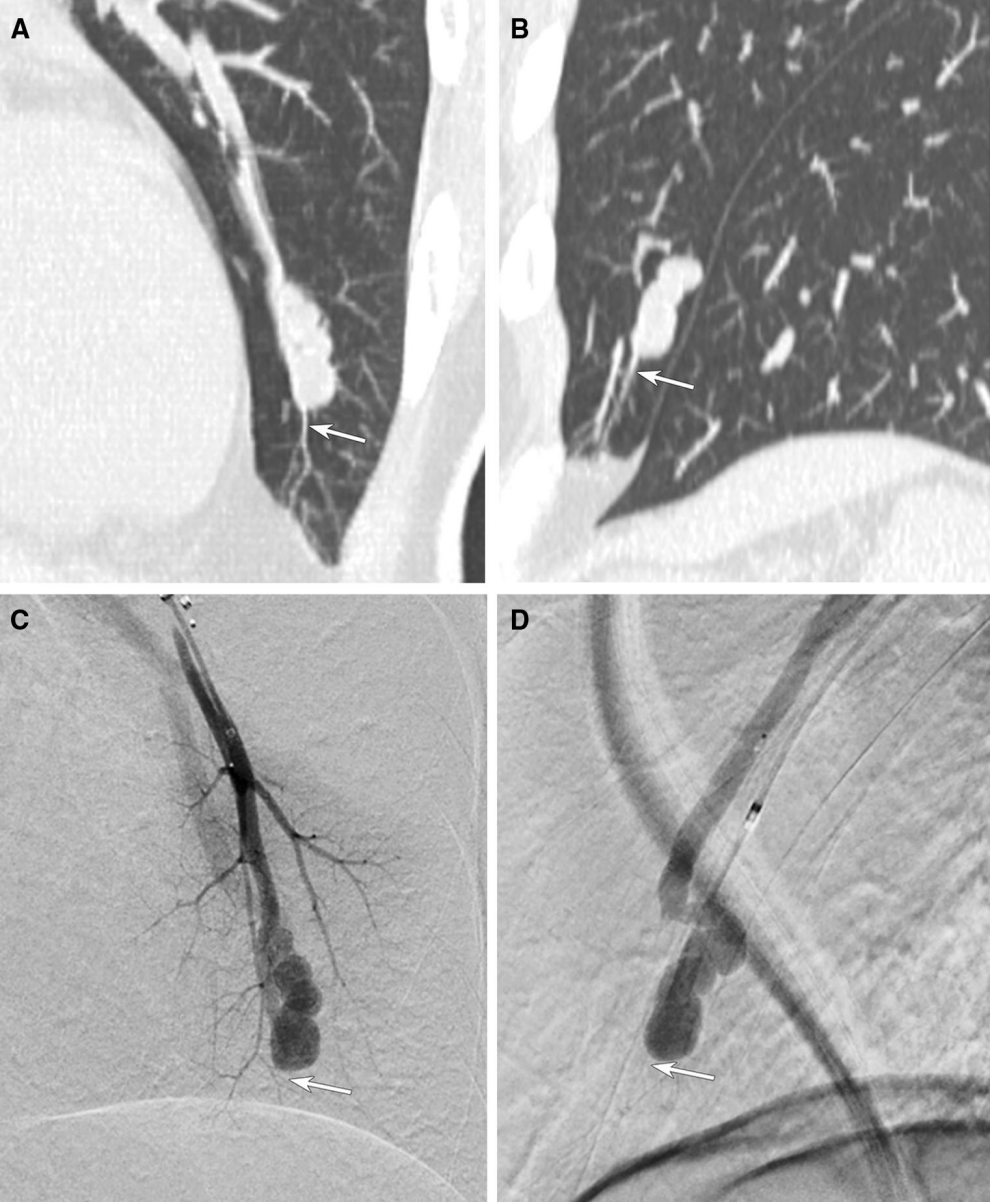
The last normal branch originating from sac cannot be visualized on selective PAG. A, B Oblique coronal (A) and sagittal (B) MPR CT images show the last normal branch (arrow) originating from sac of PAVM. C, D Frontal (C) and lateral (D) images of selective PAG cannot demonstrate the last normal branch detected on MDCT (arrow)
